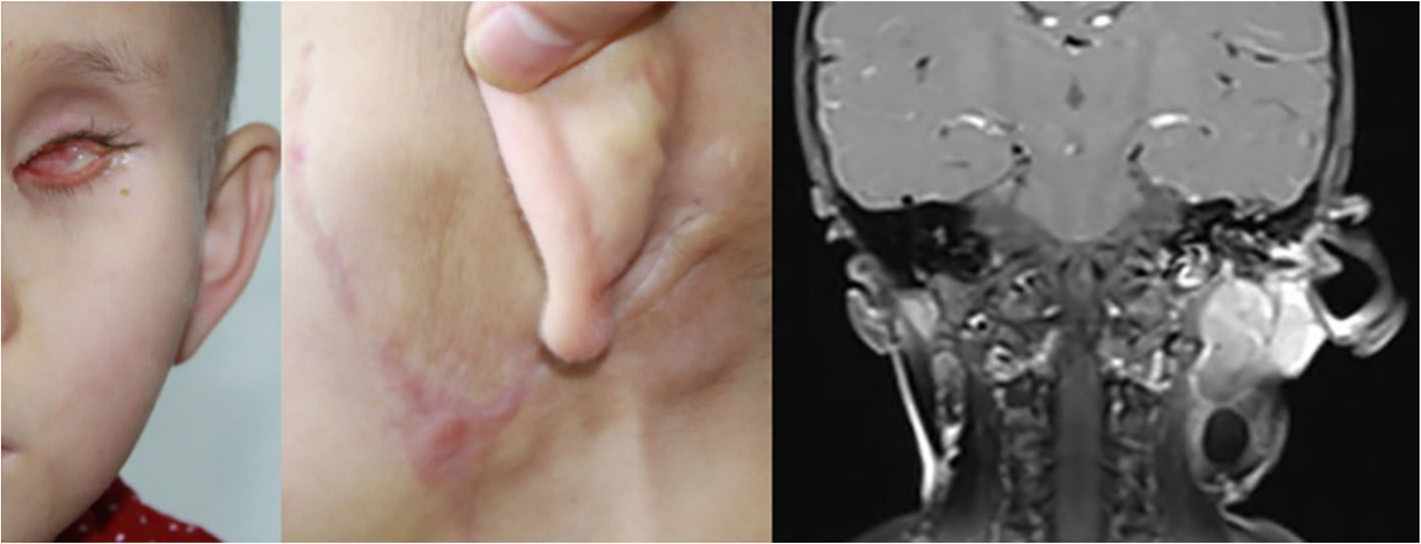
(Oct. 2016) Clinical photograph of the girl after treatment. MRI scan shows a small residual tumour in the left side of the neck. The mass seems to be hyperintense on T1 images
